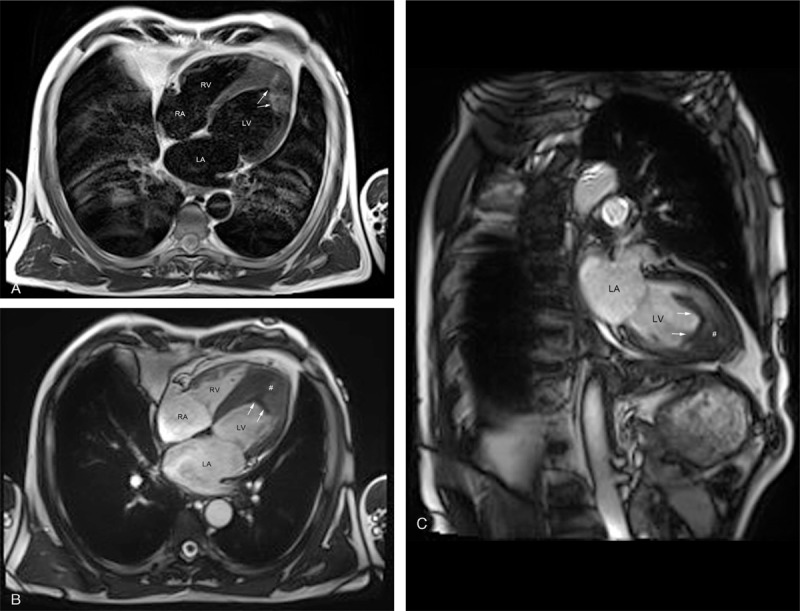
MRI images of black-blood TSE and bright-blood True FISP sequences of the first patient. (A) shows T_2_WI images with TSE sequence (white arrows indicate focal high-density signals; scan parameters, TR = 700 ms, TE = 30 ms, slice thickness = 5 mm, field of view = 340 × 340 mm, and flip angle = 180° for T_1_WI and TR = 800 ms, and TE = 77 ms for T_2_WI). (B) and (C) show single images of cine-MRI in the LV 4-chamber and 2-chamber long axis plane with the True FISP sequence, respectively (white arrows indicate the borders of the thrombus; # delineates the thickened endocardium; scan parameters, TR = 40 ms, TE = 1.4 ms, slice thickness = 6 mm, field of view = 340 × 340 mm, and flip angle = 57°). LV = left ventricular, MRI = magnetic resonance imaging, T_1_WI = T1-weighted imaging, T_2_WI = T2-weighted imaging, True FISP = true fast imaging with steady-state precession, TSE = turbo spin echo.
